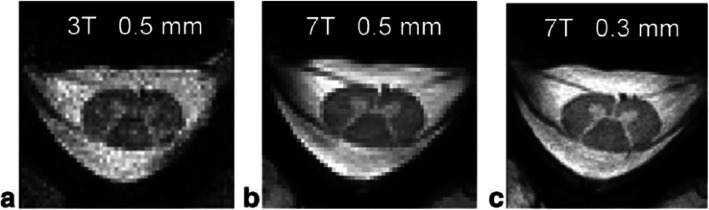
3 T *versus* 7-T MRI of C5-C6 spine in a healthy volunteer: 3-T T2 image with 0.5 × 0.5 × 3 mm^3^ resolution (a); 7-T T2 image with 0.5 × 0.5 × 3 mm^3^ resolution (b); 7-T T2 image with 0.3 × 0.3 × 3 mm^3^ resolution (c). Published with permission of the *International Society of Magnetic Resonance in Medicine* [67]
